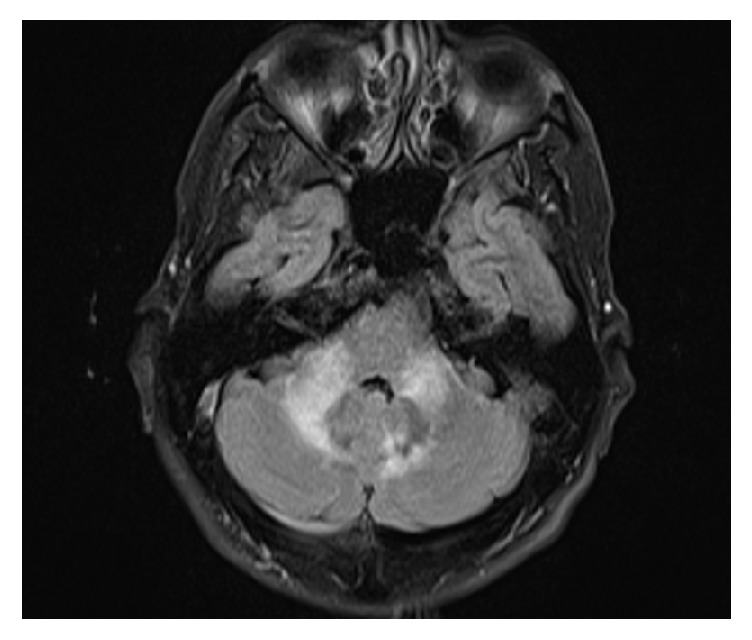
Axial T2-FLAIR MRI showing interval increase of leptomeningeal enhancement and hyperintense signal involving the left middle cerebral peduncle, cerebellar vermis, and bilateral cerebellar hemisphere.
